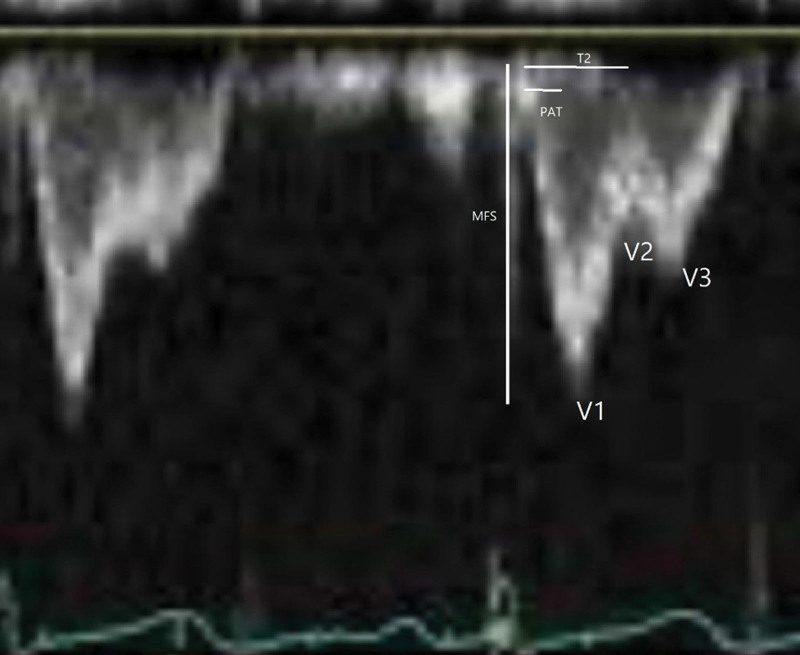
The ratio of MFS to PAT gives us PAS. MFS = maximal frequency shift, PAS = pulmonary artery stiffness, PAT = pulmonary acceleration time, T_2_ = time from onset of pulmonary flow ejection to V_2_, V_1_ = first pulmonary valve pulse Doppler velocity, V_2_ = second pulmonary valve pulse Doppler velocity, V_3_ = third pulmonary valve pulse Doppler velocity.
